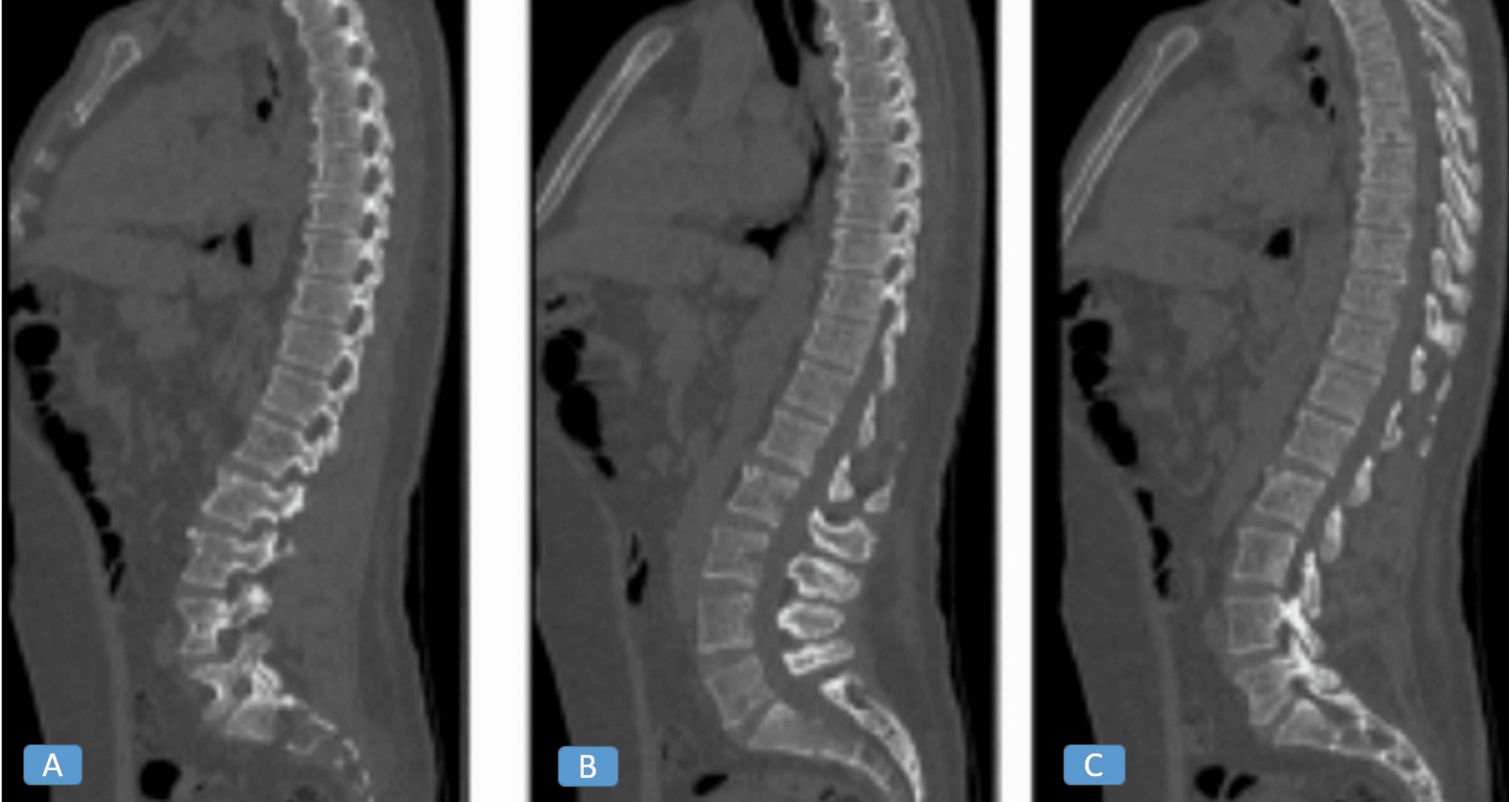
Sagittal computed tomography of the thoracolumbar spine Showing preserved vertebral alignment, normal vertebral body morphology, and a patent spinal canal without evidence of fracture, destructive lesions, or extradural masses. Reconstructions are displayed in (A) bone window, (B) soft tissue window, and (C) myelographic reconstruction.
